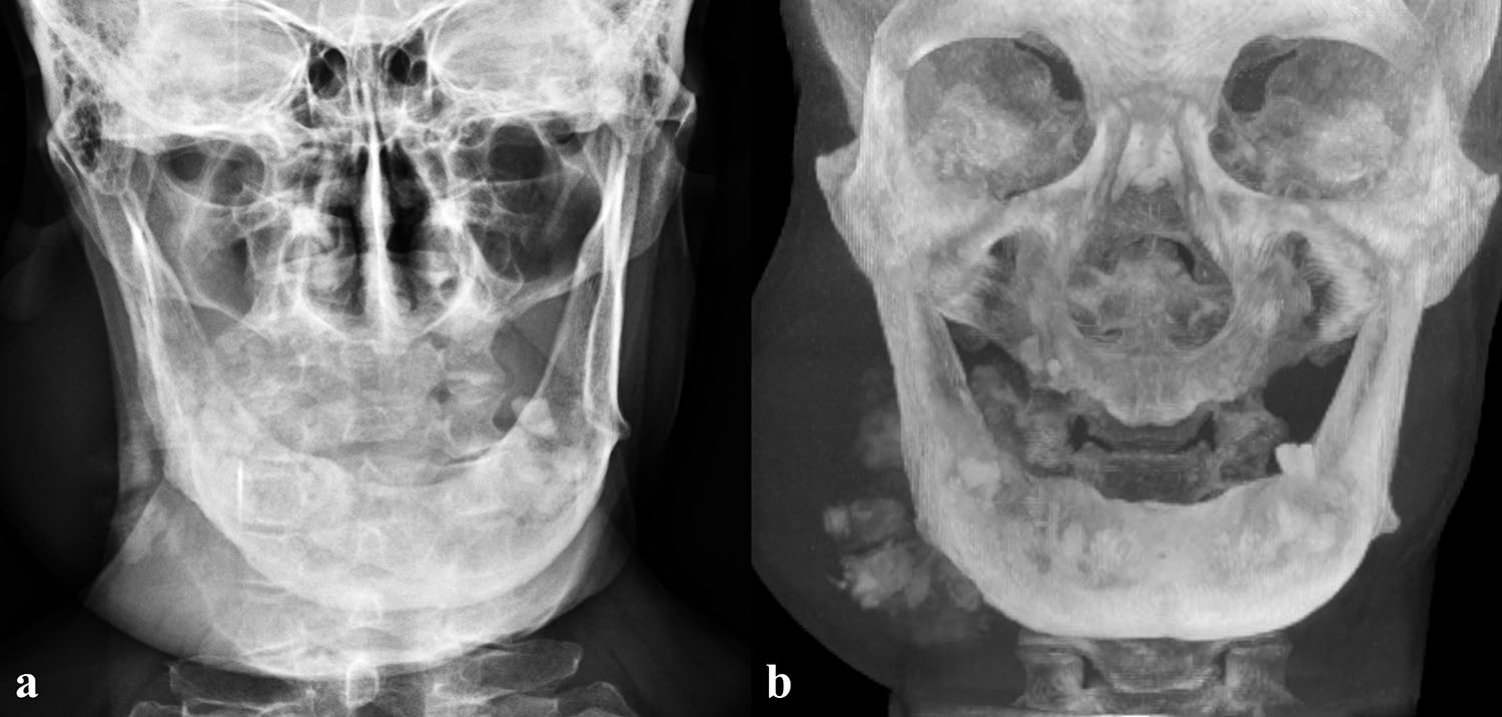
a Posterior–anterior mandible shows a sunray appearance at the right body of the mandible and a soft tissue outline of the swelling. b CBCT maximum intensity projection shows osseous internal structure of the lesion
